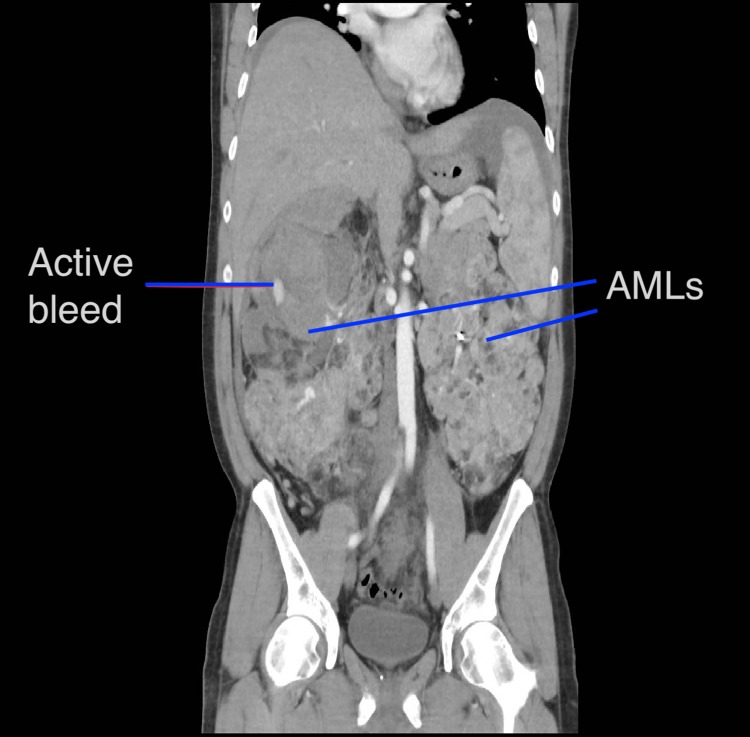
CT showing massive bilateral AMLs and hematoma formation on right upper pole. AML, angiomyolipoma.
